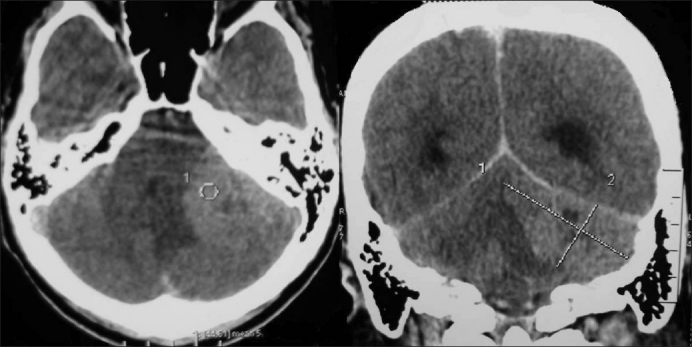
Contrast Computed tomography scan of the brain axial and coronal reconstruction showed a left CP angle mixed-density nonenhancing lesion with broad-based tentorial attachment
